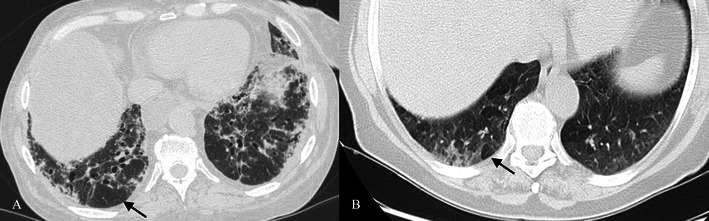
HRCT features of NSIP. NSIP patterns are shown in a 52-year-old woman (A) and in a 75-year-old woman (B). Bilateral patchy ground-glass areas, predominantly located in the middle and lower areas, represent the most important HRCT feature of NSIP. However, when the ground-glass area is not extensively distributed through the lungs, the differential diagnosis from the UIP pattern remains very difficult. Subpleural areas not involved by ground-glass opacifications (black arrows) have been suggested as other CT features helpful for diagnosis [30]
